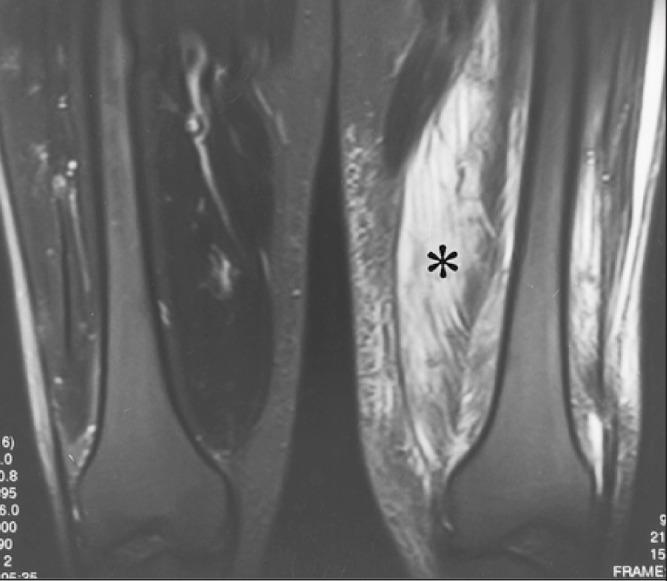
Magnetic resonance imaging following intravenous gadolinium revealing relative areas of serpiginous non-enhancement in the left vastus medialis and vastus intermedius indicative of necrosis
